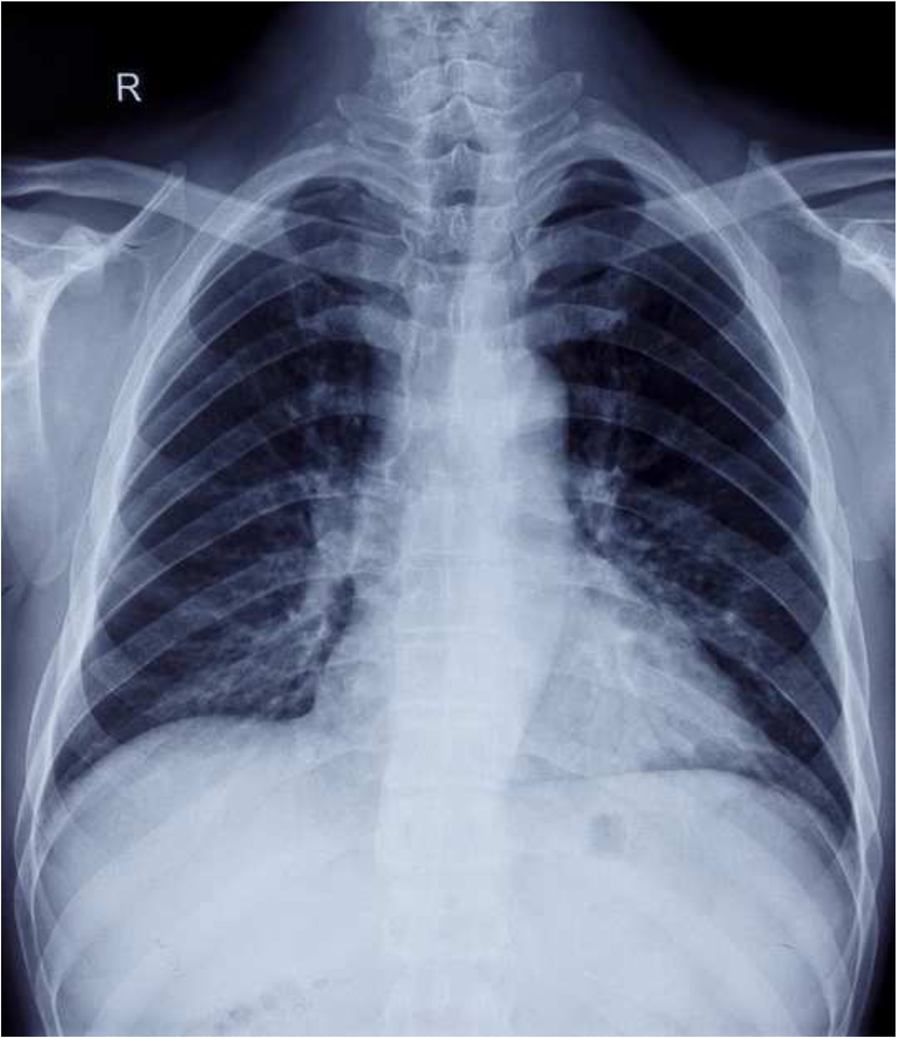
Posteroanterior chest X-ray image one week before the patient’s transfer to the respiratory intensive care unit (RICU). No significant finding was observed on the X-ray image at this date (December 2015)
